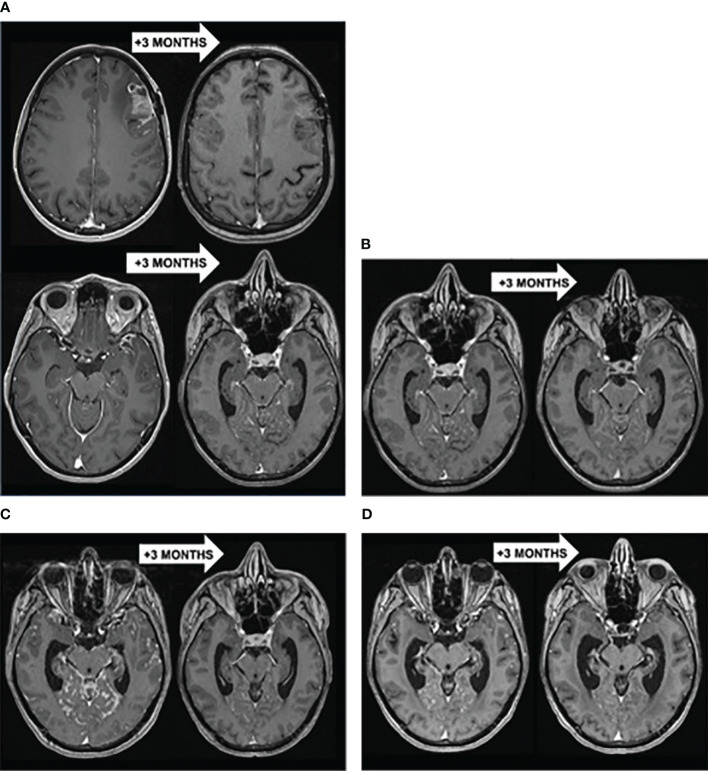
(A) Brain T1-WI MRI showing a PR of the left frontal lesion but progression of other LM lesions to stereotactic radiation therapy (5*6 Gy) followed by paclitaxel-trastuzumab-pertuzumab. (B) Brain T1-WI MRI showing a diffuse PR of LM to intra-CSF MTX (3*15 mg) followed by intra-CSF trastuzumab (150 mg) with concomitant IV pertuzumab. (C) Brain T1-WI MRI showing a diffuse PR of LM to WBRT (10*3 Gy) with concomitant IV trastuzumab-emtansine. (D) Brain T1-WI MRI showing a diffuse PR of LM to IV trastuzumab-deruxtecan.
